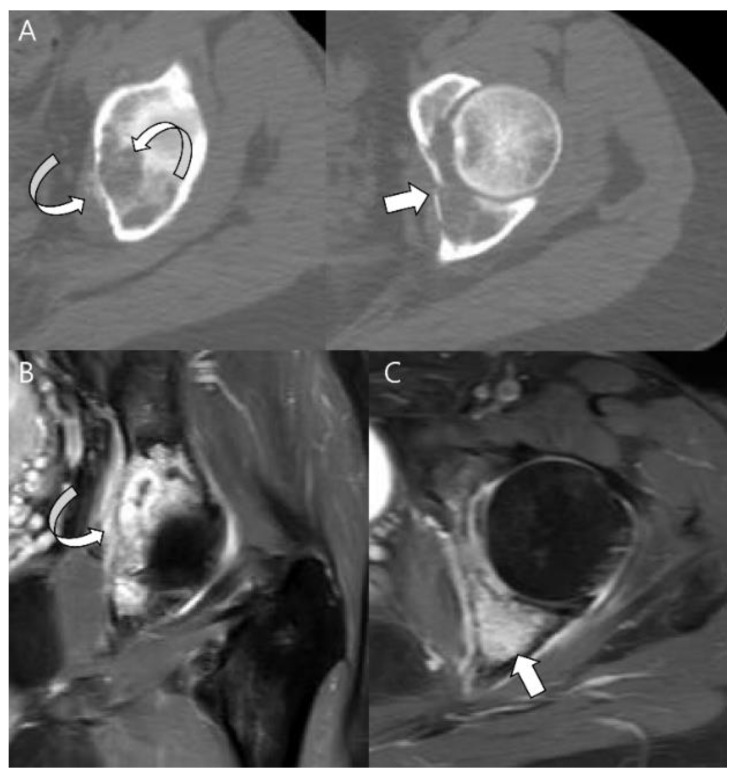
High-grade conventional chondrosarcoma of the acetabulum in a 49-year-old woman. (A) Axial CT scans reveal cortical breakage (thin arrow) with extraosseous extension containing matrix mineralization (curved arrow) in the left acetabulum. (B) Axial and (C) axial T1-weighted enhanced images with fat suppression show diffusely enhancing intraosseous (arrow) and extraosseous tumor components (curved arrow).
